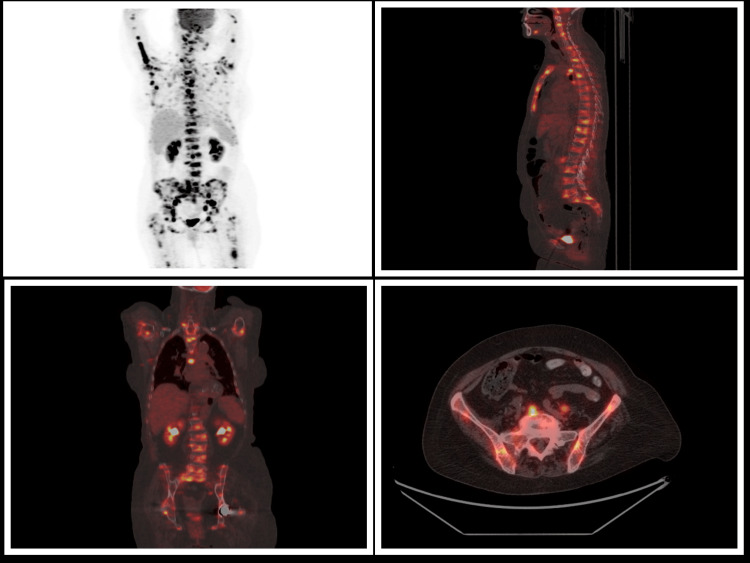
PET/CT shows pathologic uptake in the skeletal system and in multiple lymph node regions PET/CT: positron emission tomography/computed tomography
